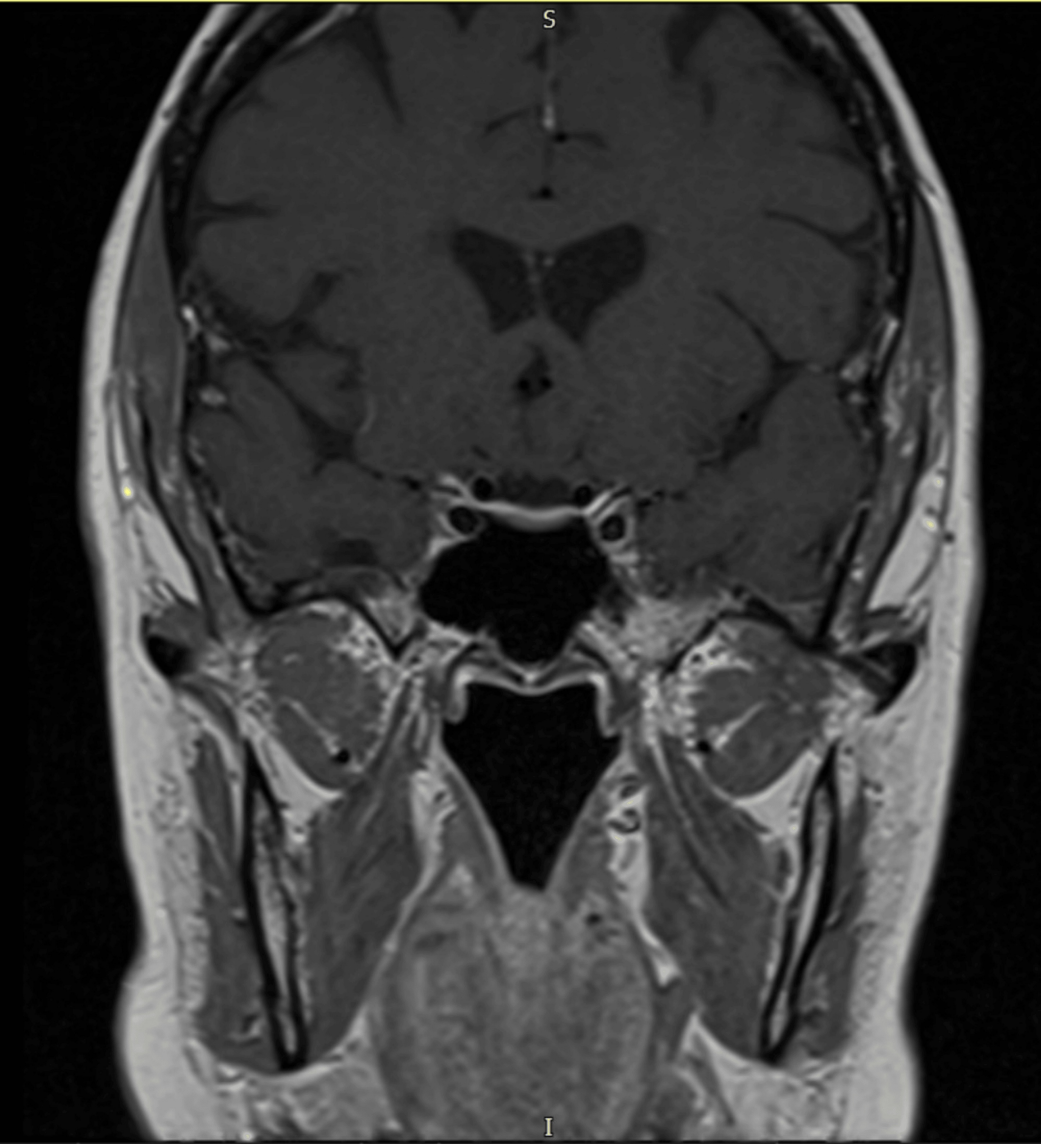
Coronal view of the pituitary MRI showing normal appearance MRI: magnetic resonance imaging
